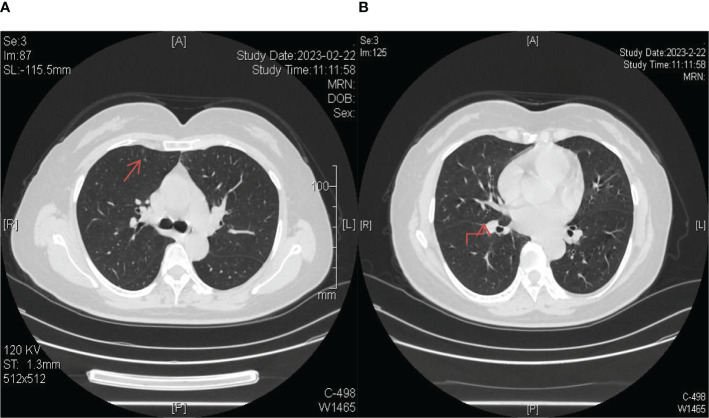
Longitudinal monitoring over a span of 3 years revealed consistent disease stability, as evidenced by regular chest CT scans. (A) The lesion in the upper lobe of the right lung; (B) The lesion near the oblique fissure in the lower lobe of the right lung.
